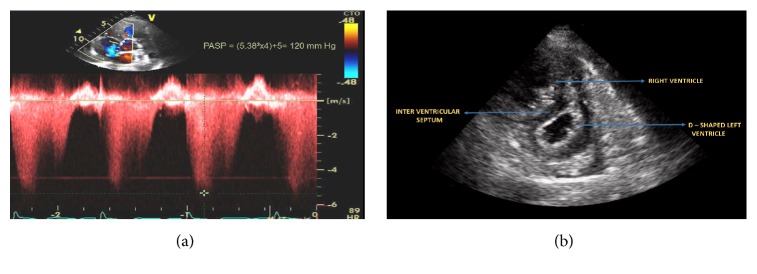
(a) Tricuspid regurgitation signal on echocardiogram at 25 weeks of gestation showing peak velocity of 5.38 m/s and Pulmonary Artery Systolic Pressure (PASP) of 120 mm Hg. (b) Echocardiogram at 25 weeks of gestation showing enlarged right ventricle and D-shaped left ventricle.
